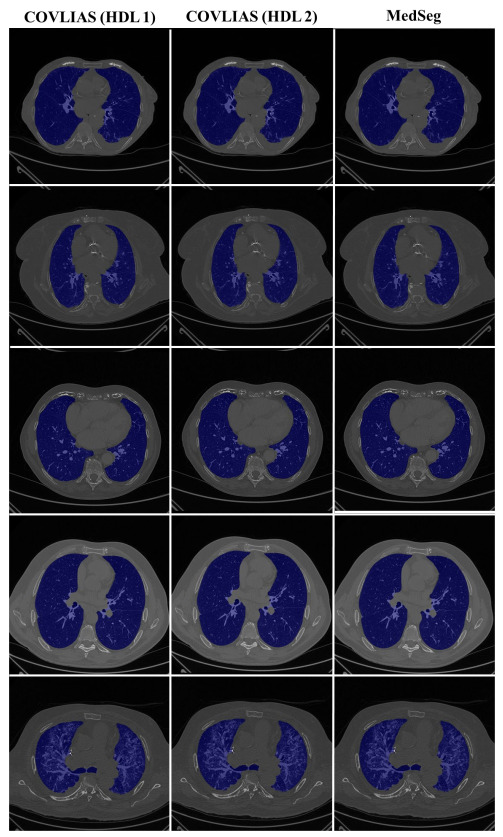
COVLIAS vs. MedSeg: Segmented mask (blue) on the non-COVID CT lung image (large lung).
